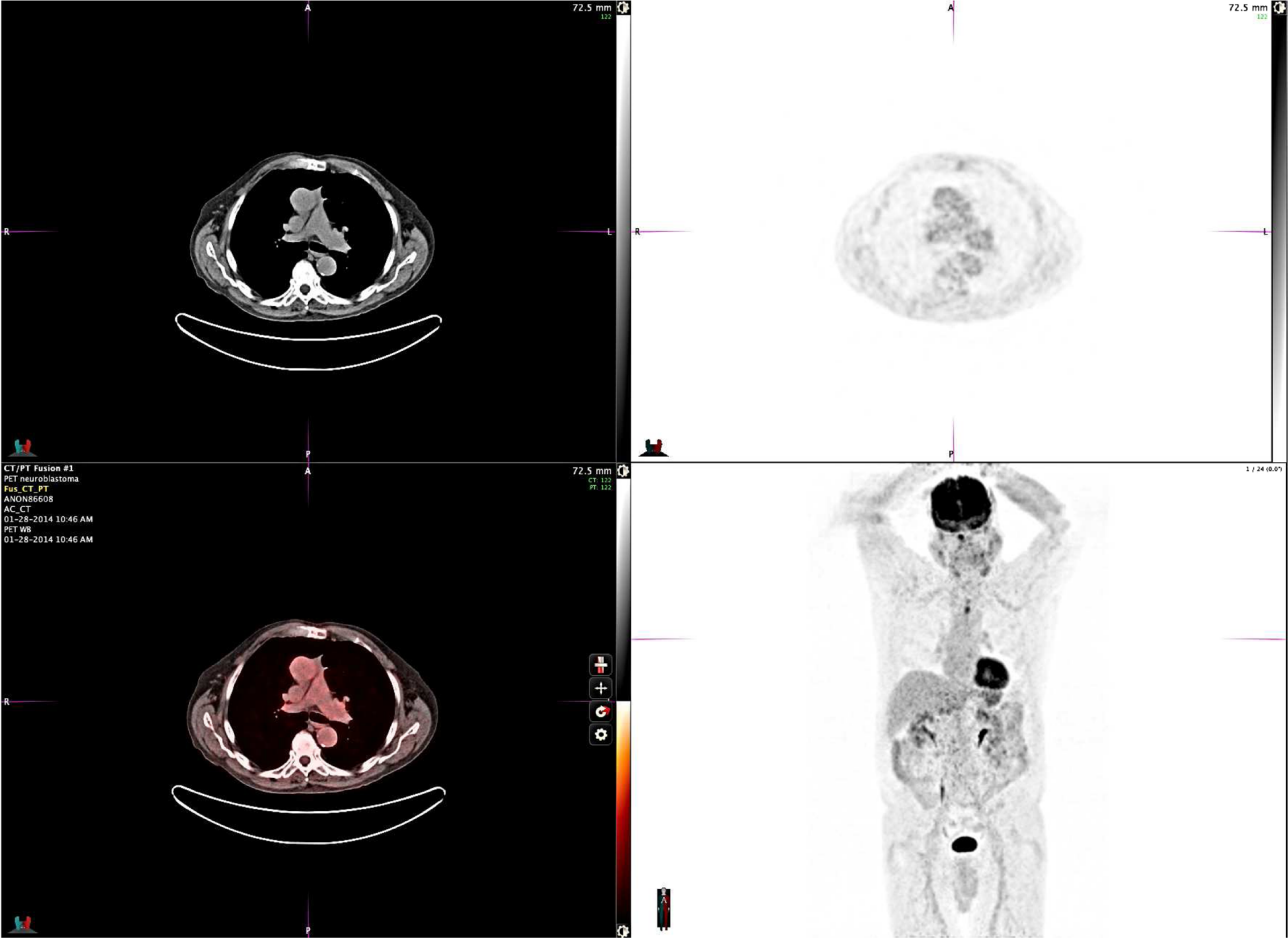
Body PET scan post-surgery. PET scan of the chest and body performed 7 months after surgical resection reveals no foci of abnormal FDG avid activity within the chest, no pulmonary nodules, and normal cardiomediastinal silhouette appearance.
